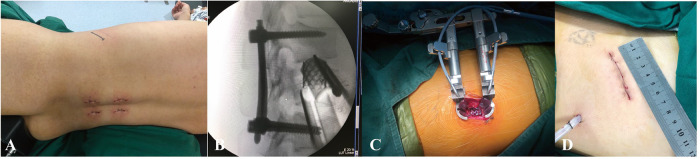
Operating room images demonstrating the AIPA surgical procedures. (A) Anterior incision of AIPA after posterior pedicle screw fixation. (B) Lateral radiograph demonstrating anterior titanium cage placement. (C) Position of the retractor system and the extent of exposure obtained. (D) Length of the anterior incision.
